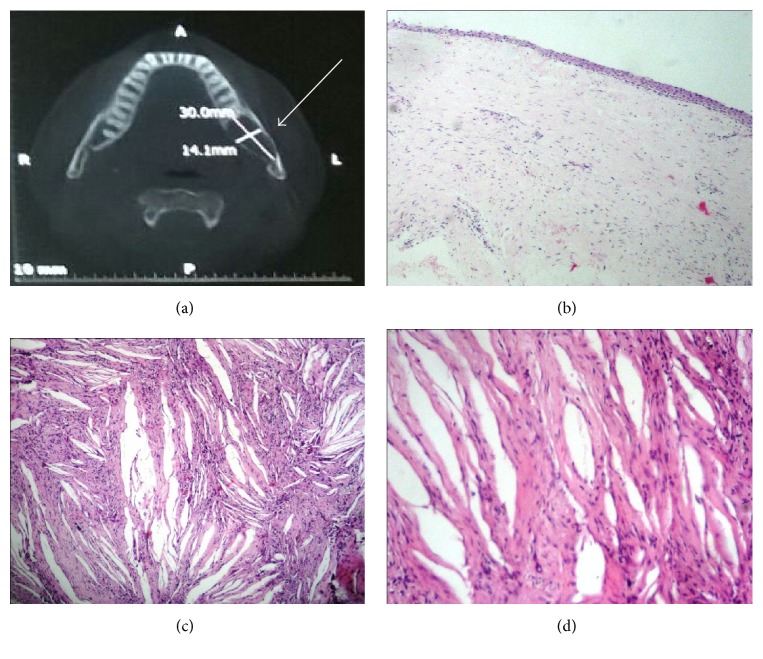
(a) CT scan revealed a well-defined radiolucency (30 × 14 mm in size) present on left angle of mandible region; (b) on incisional biopsy, 2-3-cell layer thick nonkeratinized stratified squamous cystic epithelial lining which showed proliferation in few areas associated with underlying inflammation (H/E 4x); (c) on excisional biopsy cholesterol clefts in cystic wall (H/E 10x); (d) cholesterol clefts in cystic wall (H/E 40x).
